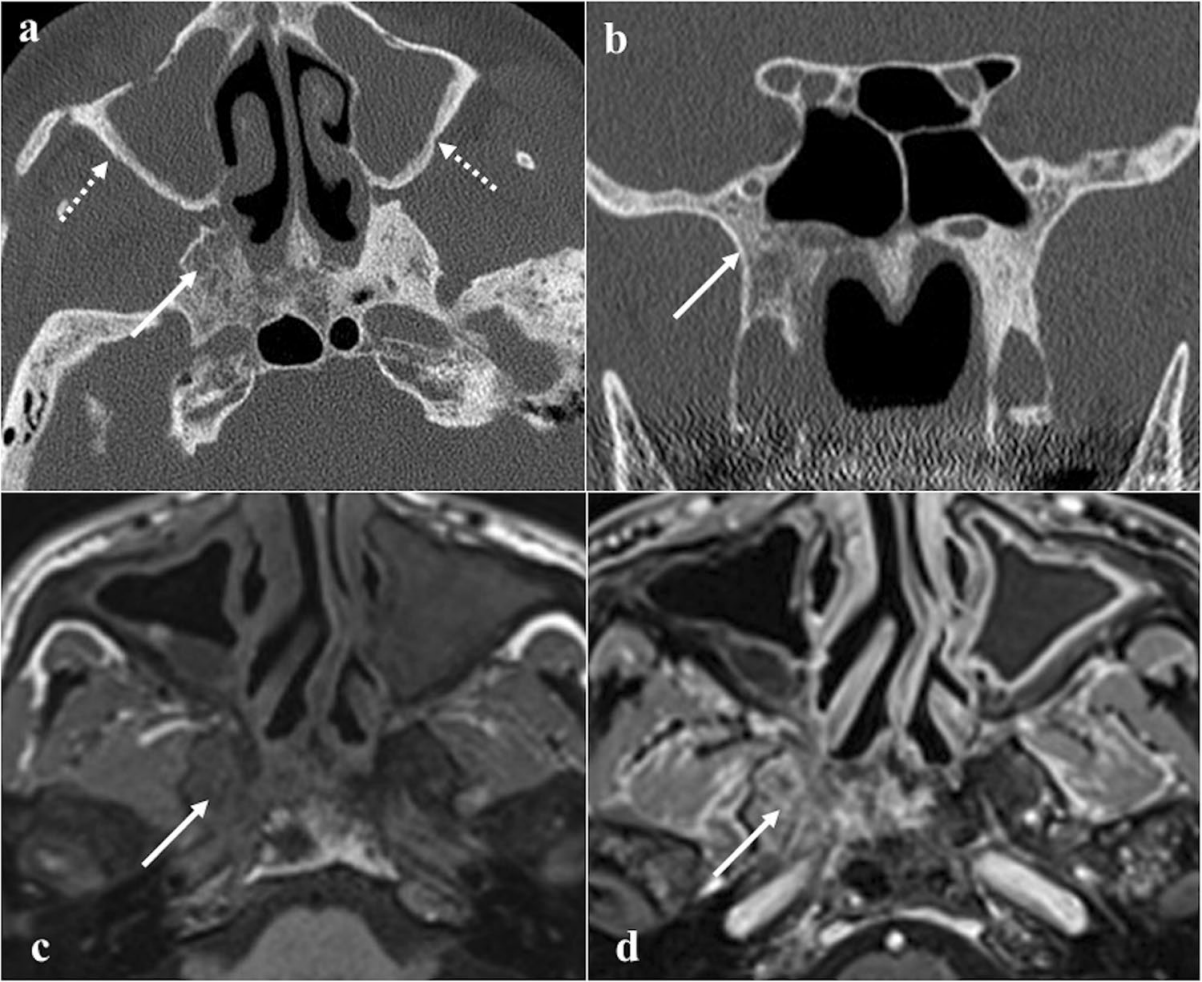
Sphenoid osteomyelitis in a 67-year-old male patient with chronic rhinosinusitis. Axial (a) and coronal (b) CT with bone algorithm reconstruction show maxillary sinusitis, osteolysis of the right greater sphenoid wing (white arrows) without cortical involvement, and thickening of maxillary sinus walls on both sides (dotted white arrows). Axial MRI images show inflammatory bony changes of the right greater sphenoid wing characterised by low SI on T1W image (c, white arrow) and mild enhancement after gadolinium contrast agent injection on T1W fat-saturated image (d, white arrow)
